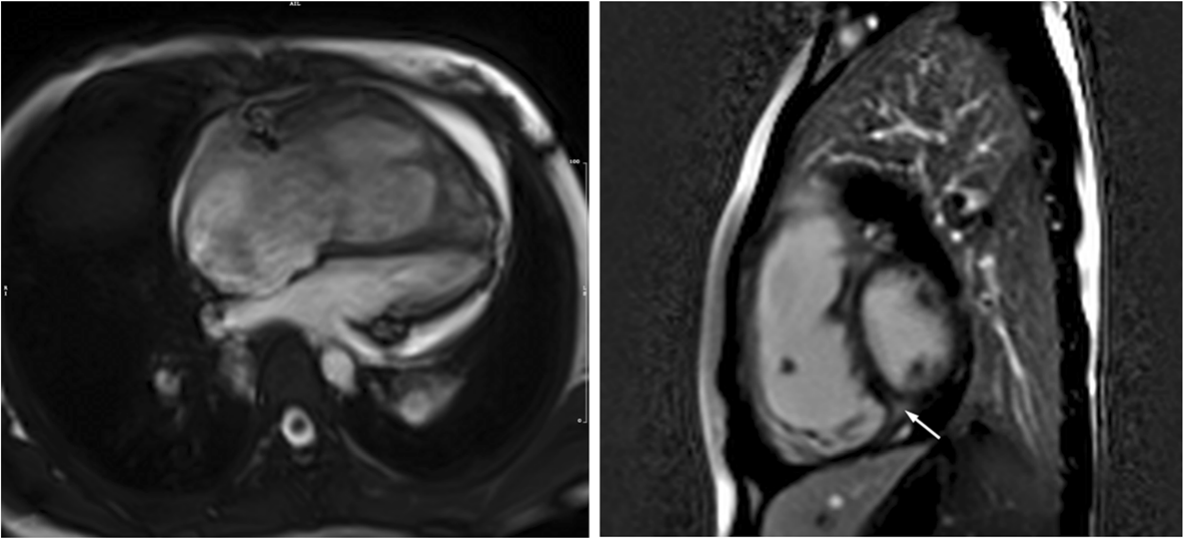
Cardiac magnetic resonance imaging confirms dilated right ventricle with late enhancement at the inferior right ventricular insertion point (arrow)
